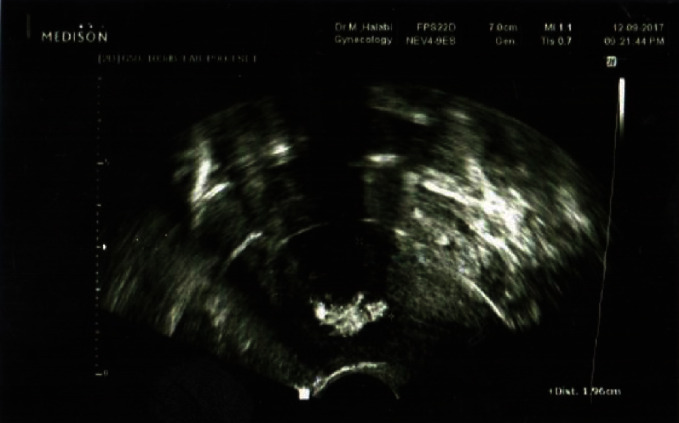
View of the uterus during abdominal ultrasonography.
